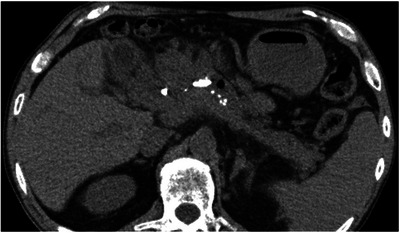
Abdominal computed tomography image at the time of consultation when the patient presented at the hospital. Atrophy and calcification of the pancreatic parenchyma associated with chronic pancreatitis were noted. The fat levels surrounding the pancreas remained unchanged.
